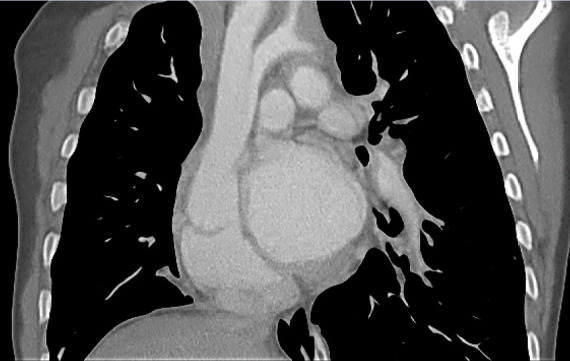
Computed tomography with coronal view: A severe hypoplasia of the distal aortic arch is observed. The persistent ductus arteriosus is visualized as a vascular connection between the left pulmonary artery and the descending aorta.
